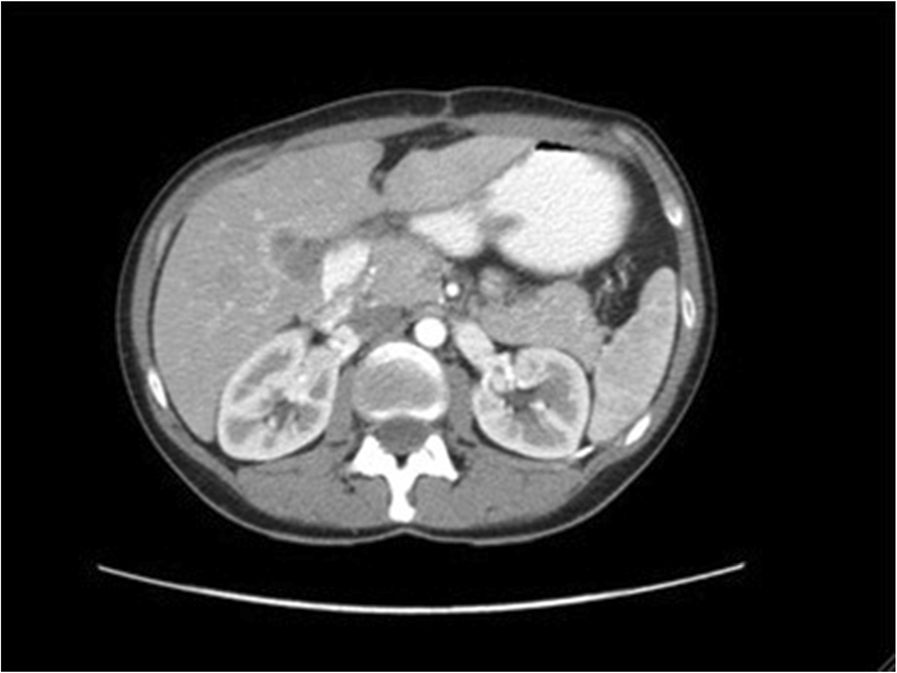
CT image showing the volume of the left liver lobe immediately after the right hepatectomy and free of disease (hypertrophy is evident)
